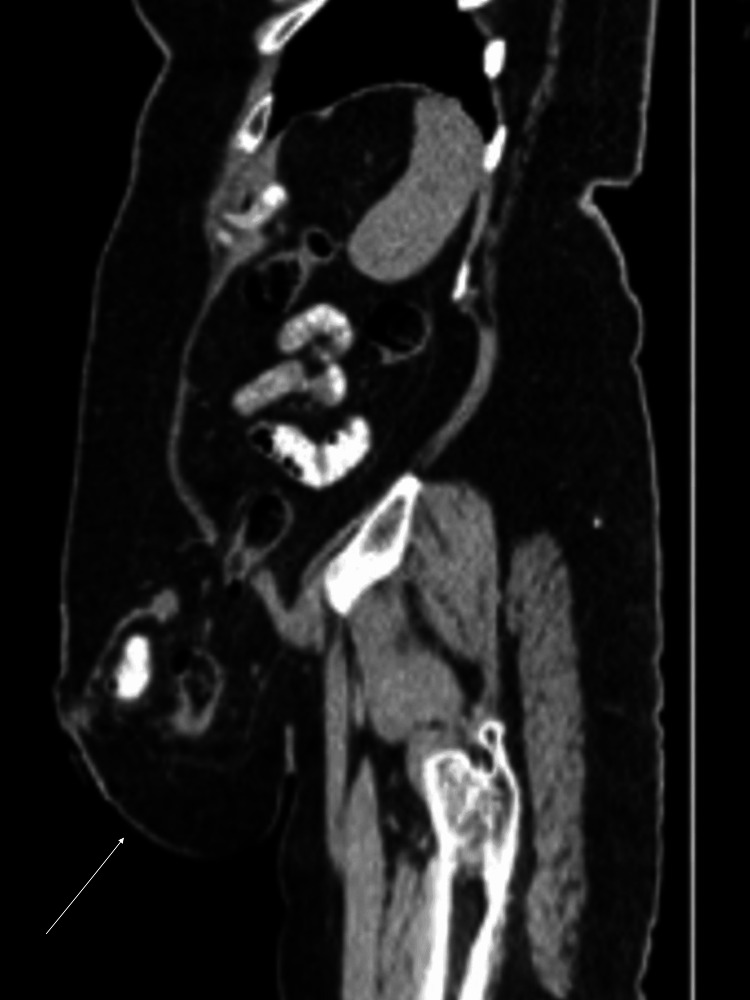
Initial CT scan at presentation showing parastomal hernia containing small bowel loops without obstruction.
